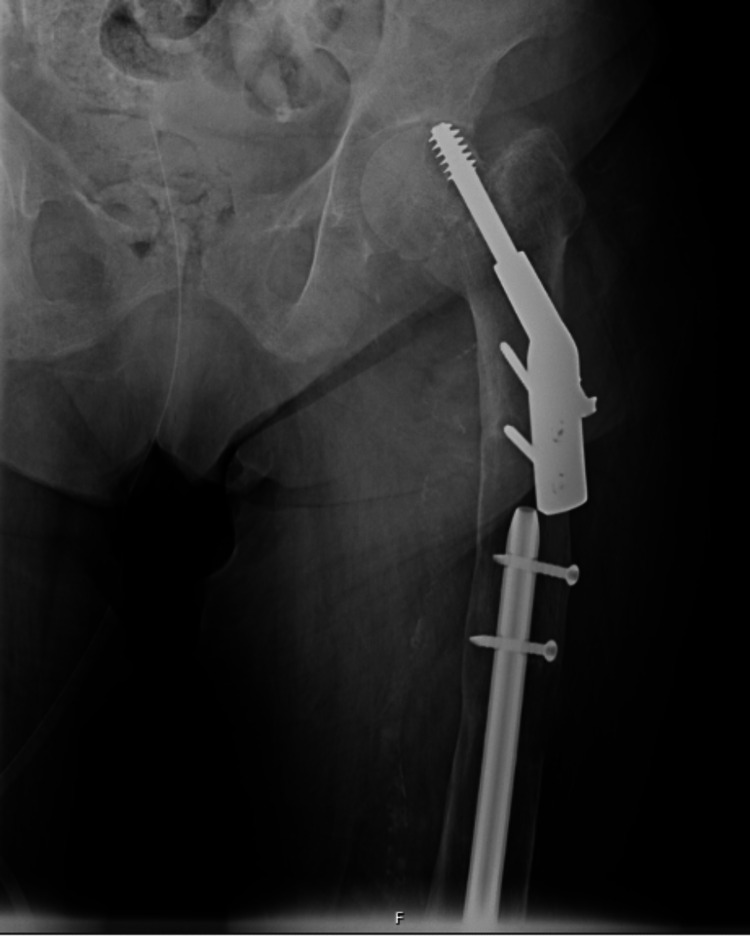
Radiograph of the left hip showing prosthetic dislocation.
